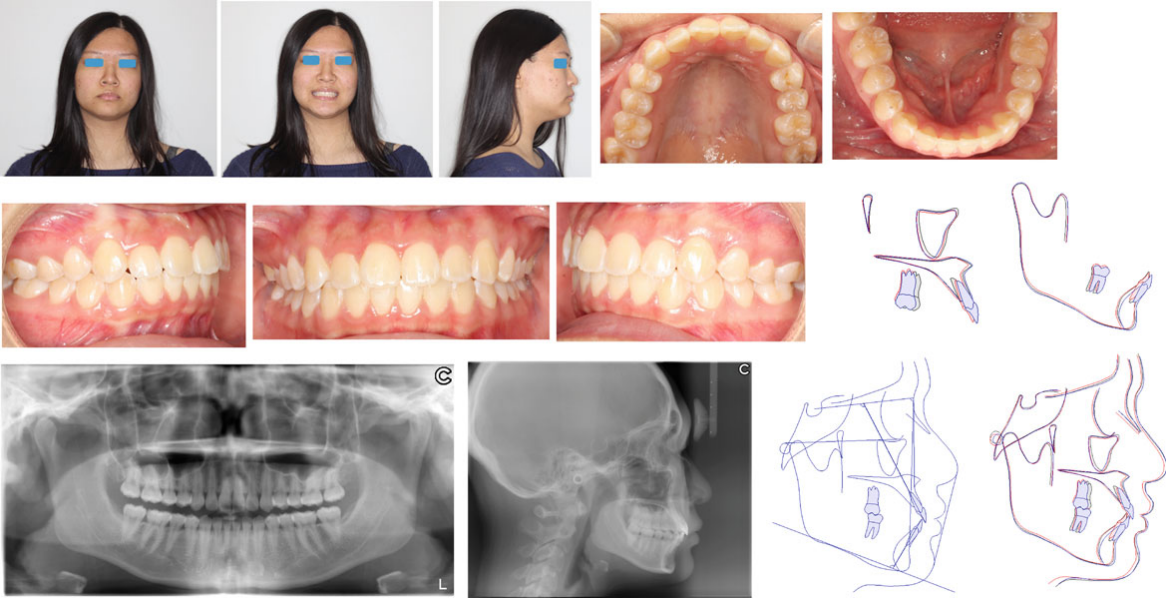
Two years posttreatment extra- and intraoral photographs, radiographs, cephalometric tracing (blue), and superimpositions of pre- (black), post- (red), and 2 years post-treatment (blue).
